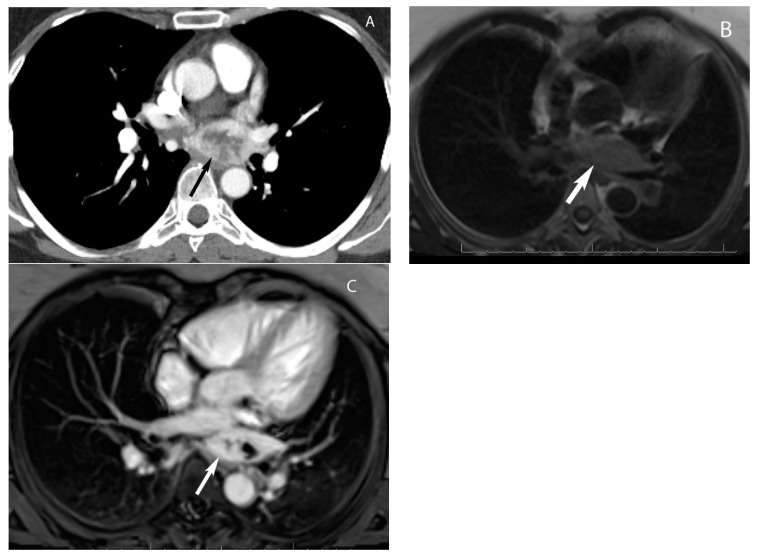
Paraganglioma. CT (A) shows a hypervascular mass with central necrosis in the visceral mediastinum behind the left atrium. MRI (B,C) shows the mass is hyperintense compared to muscle upon black blood double inversion MRI (arrow in (B)) and hypervascular upon post-gadolinium imaging (arrow in (C)).
